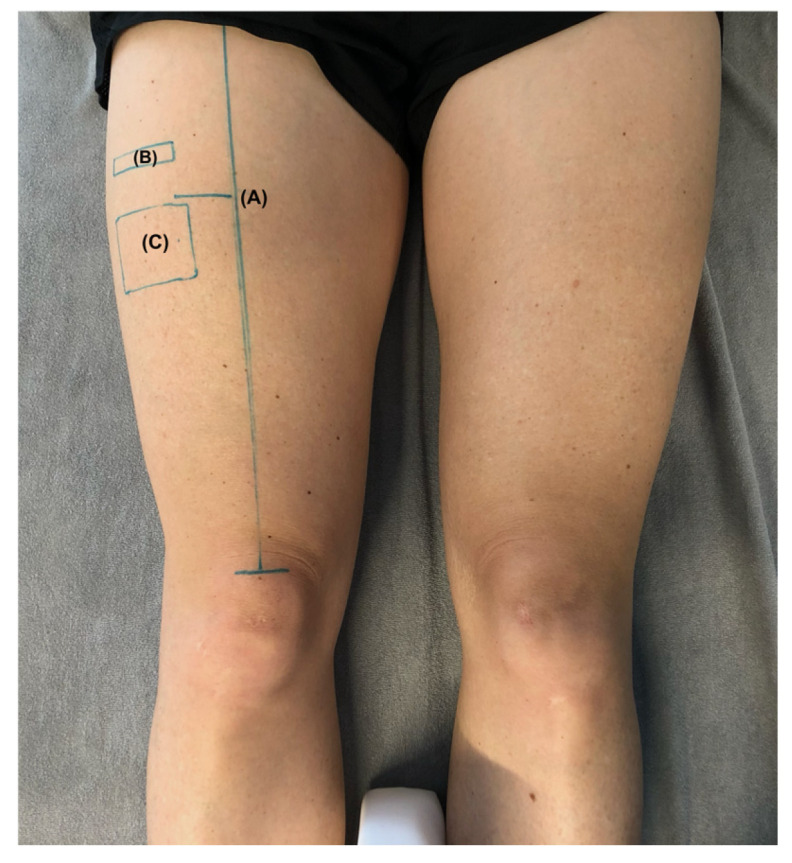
Probe placement markings for the ultrasound probe and near-infrared spectroscopy probe: (A) midpoint of the spina iliaca anterior superior and the superior border of the patella; (B) position for the ultrasound probe; (C) position for the NIRS device.
